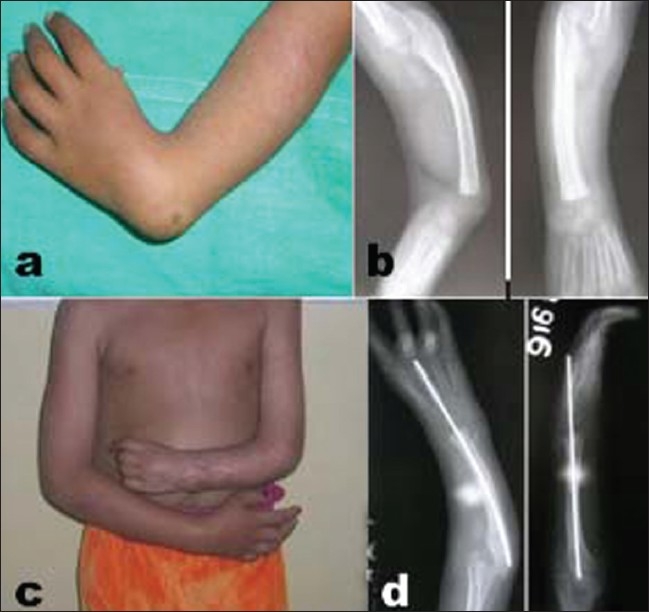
Case no 4 (a) At presentation with grade IV deformity on left side. (b) X-rays after splinting showing alignment of hand over forearm. (c) Clinical photograph at 2 years follow up after surgery showing maintenance of correction, the left forearm is short than the normal side. (d) X-rays at 2 year follow up showing retained K-wire and maintained correction
